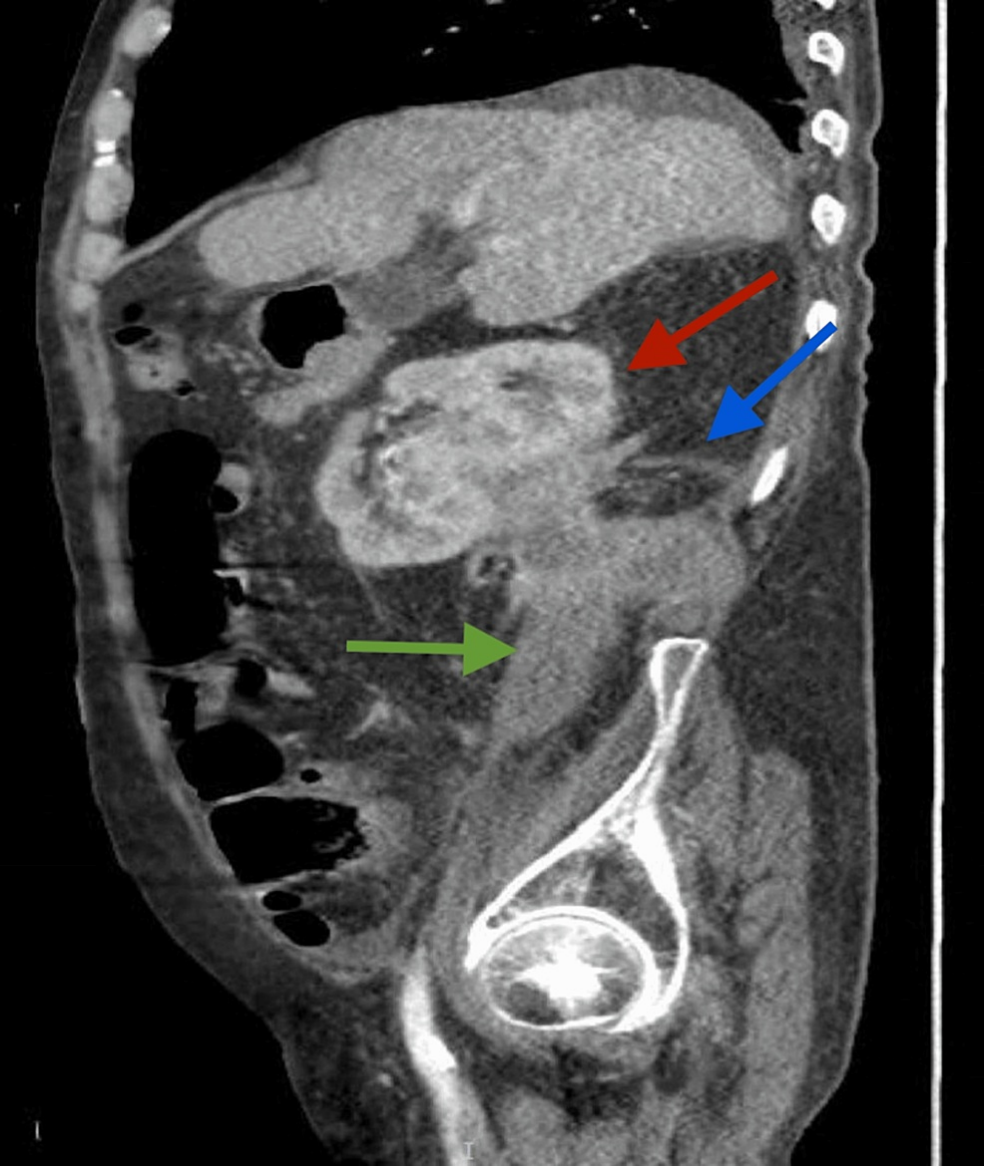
Computed tomography scan showing extensive perinephric abscess (red arrow), psoas abscess (green arrow), and perinephric cutaneous fistula to the back (blue arrow).
